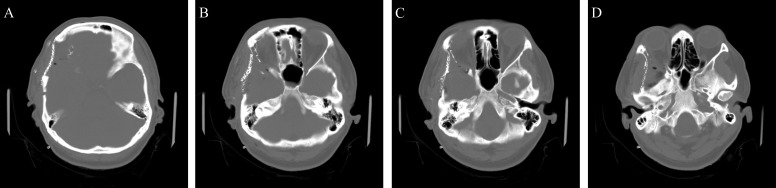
Postoperative CT following right orbitozygomatic craniotomy and cranial reconstruction
